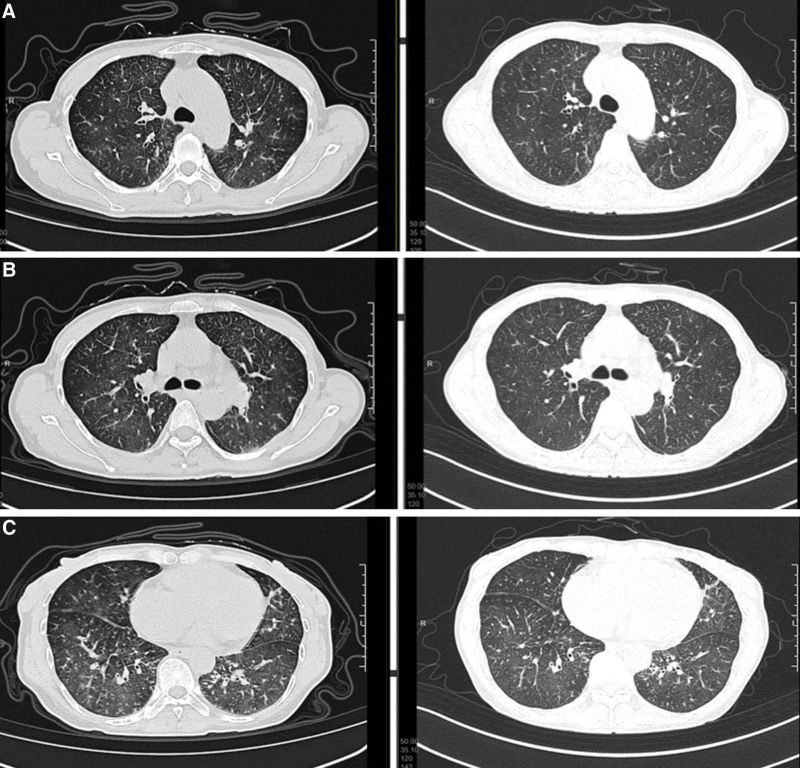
Comparation of computed tomography on day 1 and day 19.
